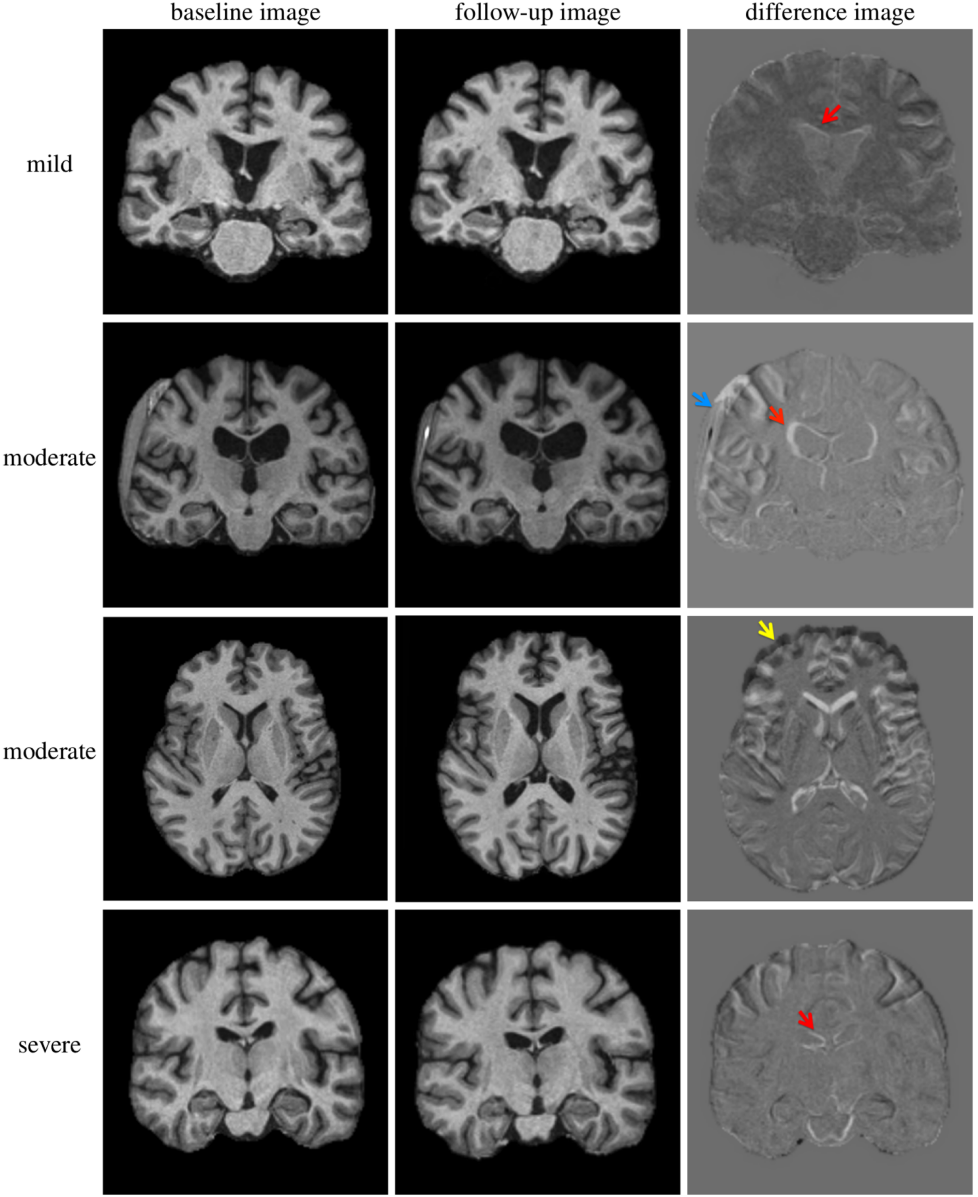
Examples of MR images of TBI patients. Four examples of T1-weighted MR images (brain extracted) of subjects from a prospective TBI cohort visualised in coronal or axial view. Top: patient with mild TBI (male, 72 years, Glasgow Coma Scale (GCS): 14, extended Glasgow Outcome Score (GOSe): 8, Marshall Classification Score (MCS): 1, cause: fall accident). Second row: patient with moderate TBI (female, 55 years, GCS: 3, GOSe: 4, MCS: 4, cause: fall accident). Third row: moderate TBI patient (male, 38 years, GCS: 11, GOSe: 5, MCS: n/a, cause: car accident). Bottom: patient with severe TBI (female, 33 years, GCS: 4, GOSe: 3, MCS: 2, cause: car accident). Left: baseline MR image acquired in the acute phase (days after the injury), Middle: follow-up MR image acquired in the chronic phase (months after the injury), Right: difference image of rigidly aligned images. Enlarged ventricles (red arrows), a subdural haematoma (blue arrow) and deformed/compressed frontal region (yellow arrow) are indicated in the difference images.
